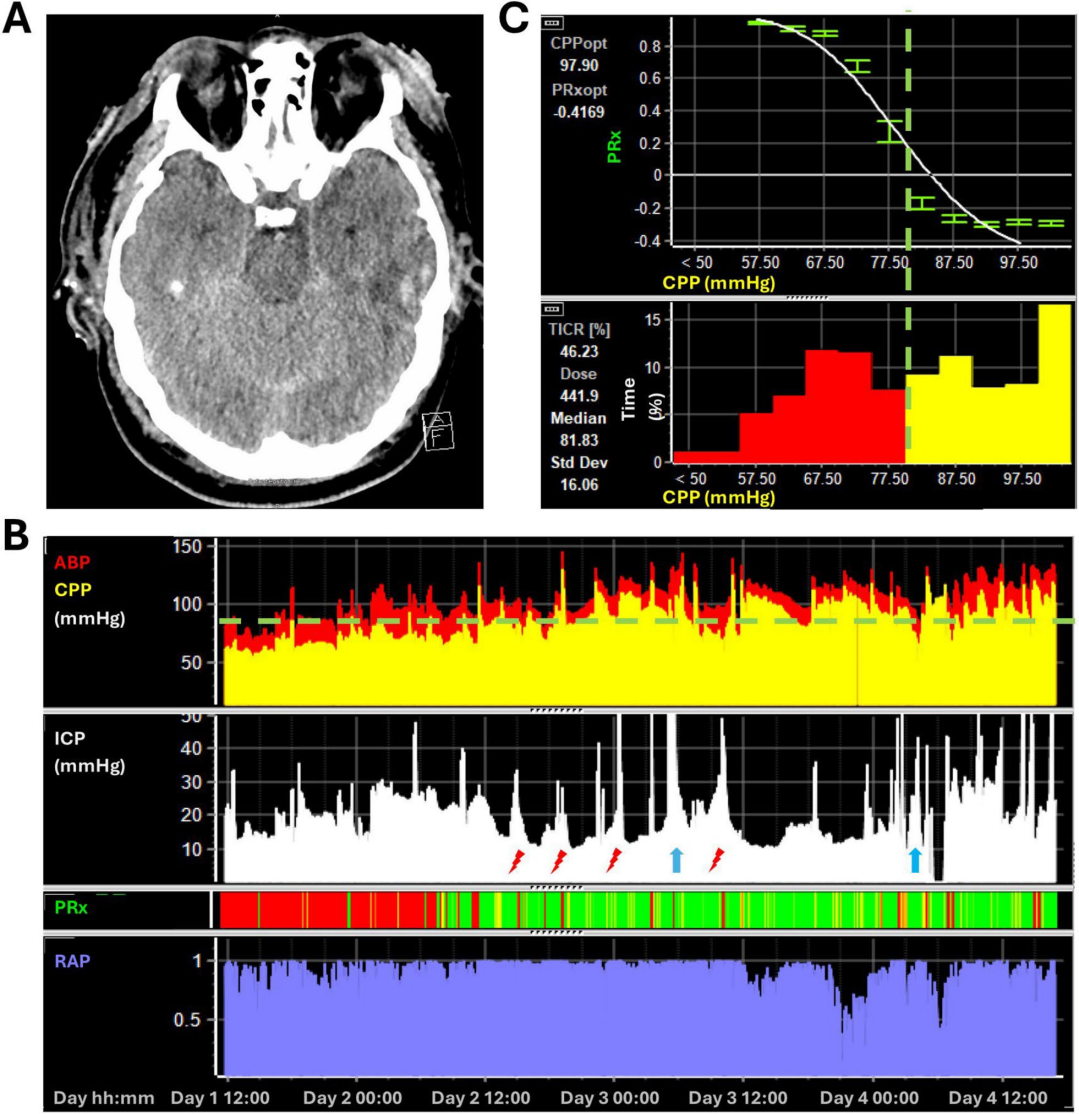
Monitoring trends and initial imaging of case 1. Panel A displays the initial CT scan with the intracranial hemorrhages. Panel B displays the MMM data. The minute-by-minute ABP, CPP, and ICP traces are shown in the upper panels. PRx is represented with a color-coded bar, where red indicates impaired and green indicates preserved vascular reactivity. The RAP, representing cerebrovascular compensatory reserve, is shown below. During the monitoring, the patient suffered multiple episodes with intracranial hypertension even in reaction to routine nursing procedures (marked using red bolts). Additionally, after an episode with high ICP (marked with the first blue arrow), the patient developed bilaterally non-reactive pupils. At the end of his stay, he developed refractory intracranial hypertension likely associated with infarction (as evidenced by the non-reactive pupils; marked with the second blue arrow). Panel C displays the relationship between CPP and PRx considering the full monitoring time and the frequencies of CPP values within the histogram below. The superimposed dashed lines in Panel B and C denote the CPP value representative of the LLR
